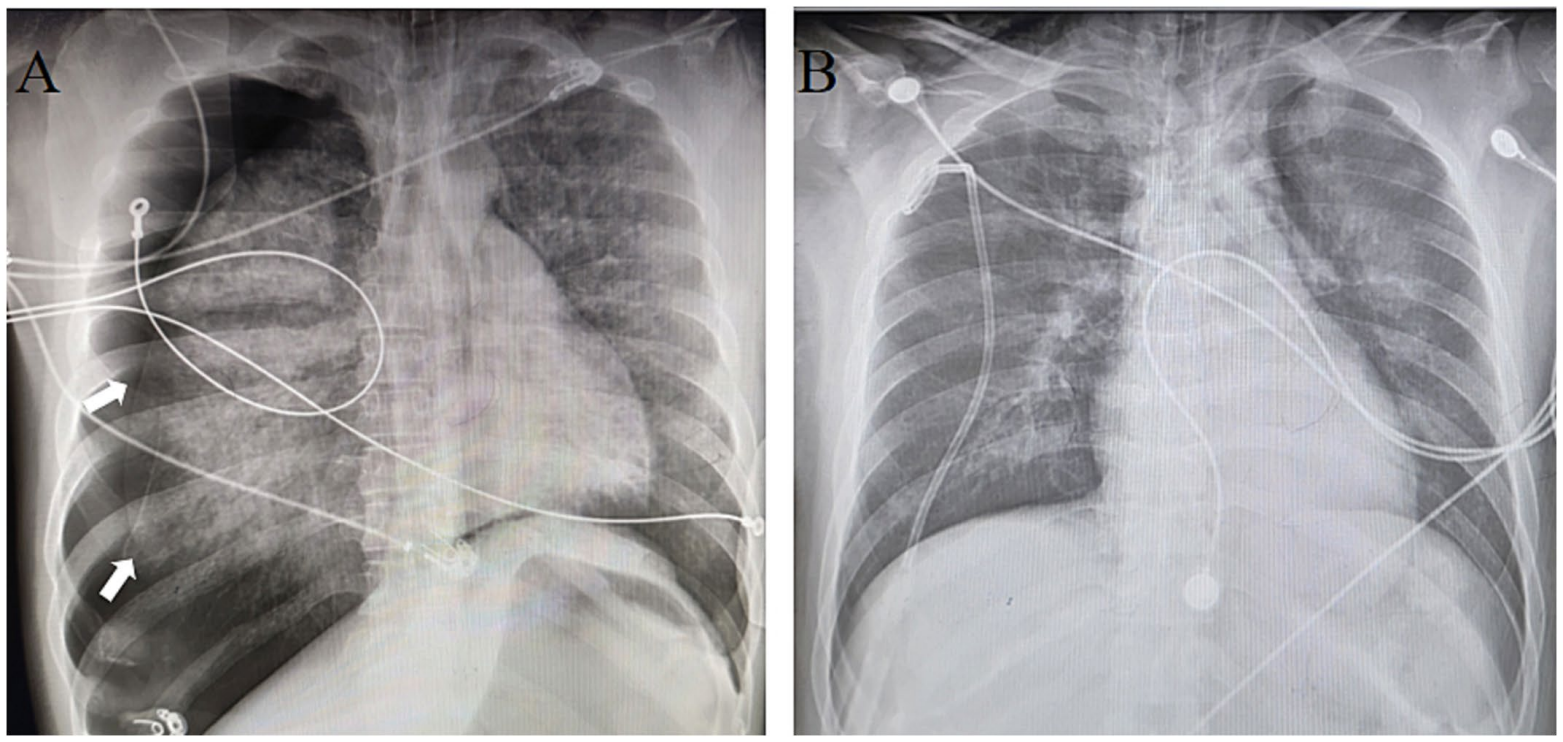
Chest X-ray. (A) Pneumothorax, white arrows: absence of lung markings. (B) Marked resolution of pneumothorax after thoracostomy tube placement.
